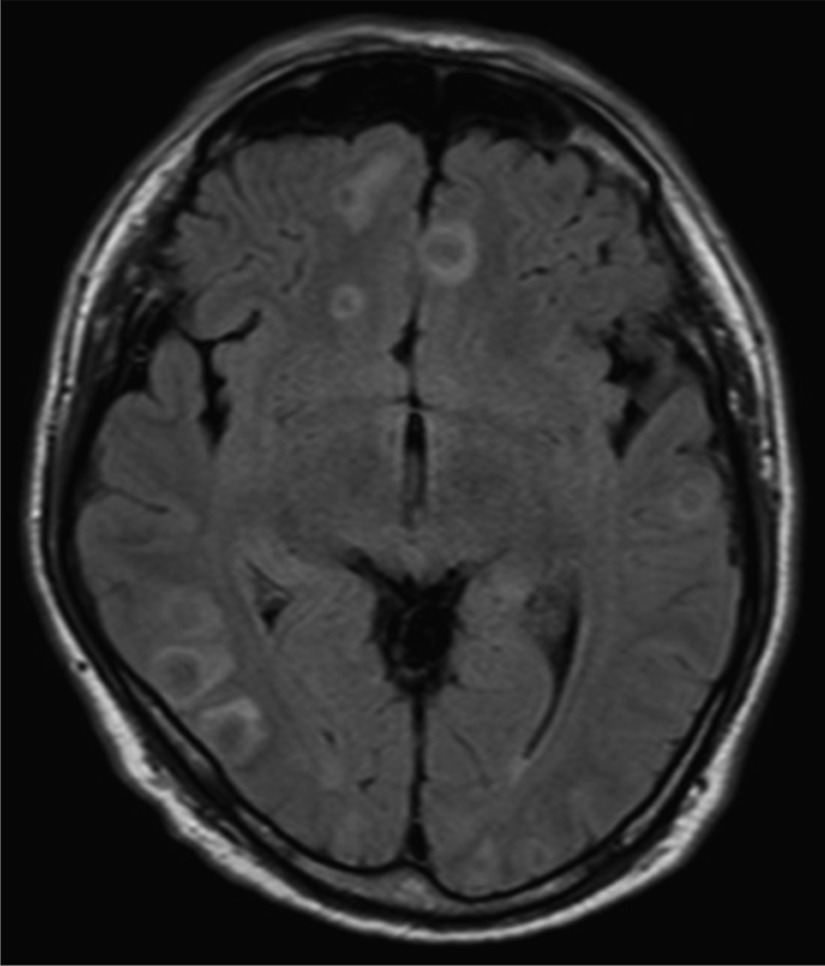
Enhanced head magnetic resonance imaging revealed multiple ring-enhancing lesions over the cerebrum and cerebellum
